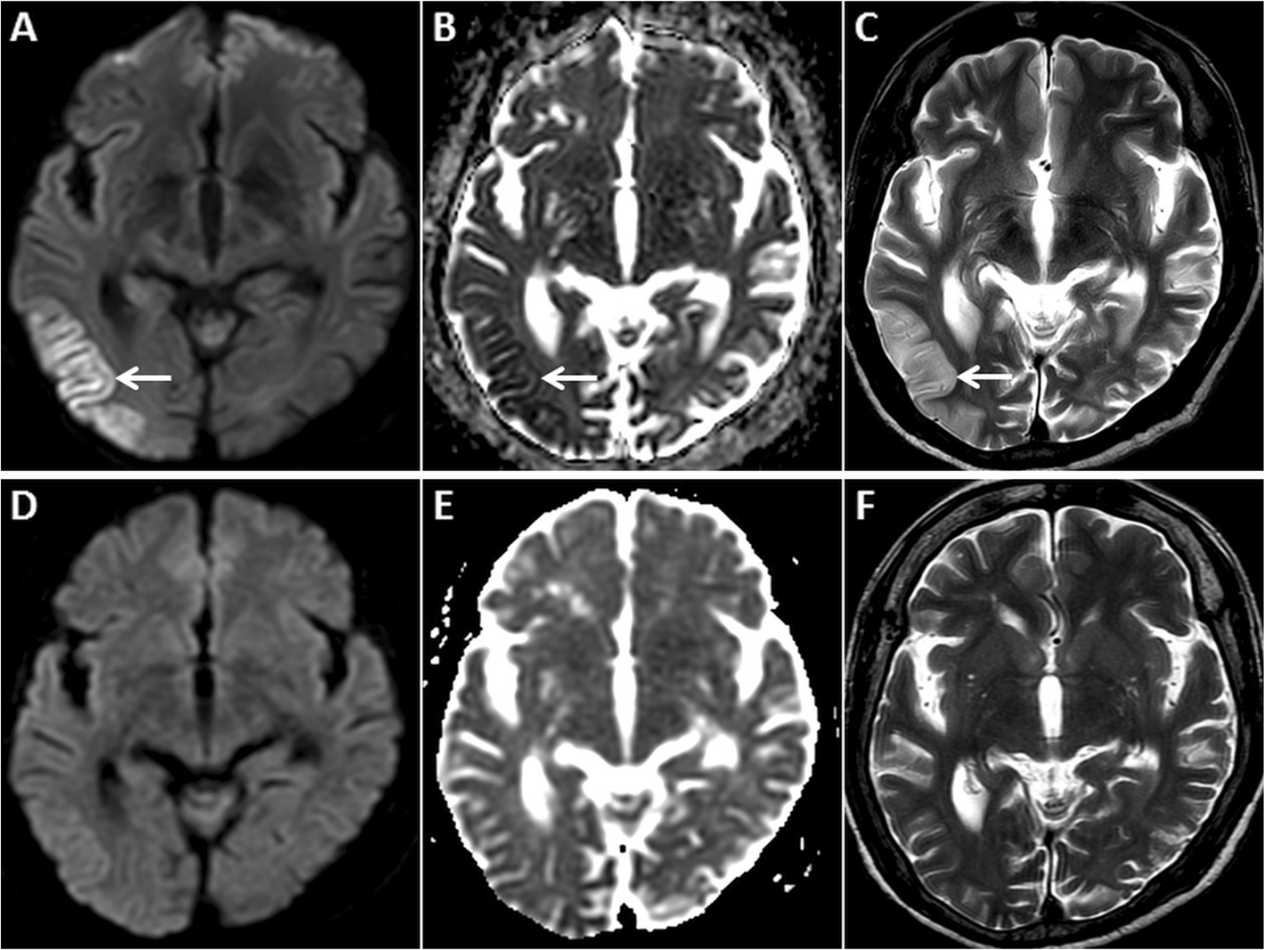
MRI of the brain in a 67-year-old female patient presenting with a seizure. GRD is observed in the right temporo-occipital region (arrow) on axial DWI (a) and ADC image (b). Also note associated cortical swelling and hyperintensity (arrow) on axial T2W image (c). Follow-up MRI after 4 days reveals complete resolution of the cortical signal abnormality and swelling on axial DWI, ADC and axial T2W images (d, e, f, respectively)
